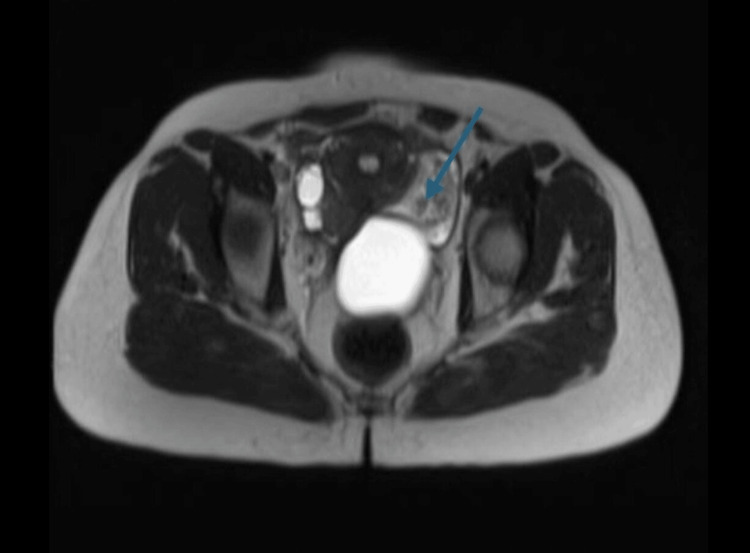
MRI showing lipoleiomyoma as a T1 hyperintense lesion (see arrowhead).
